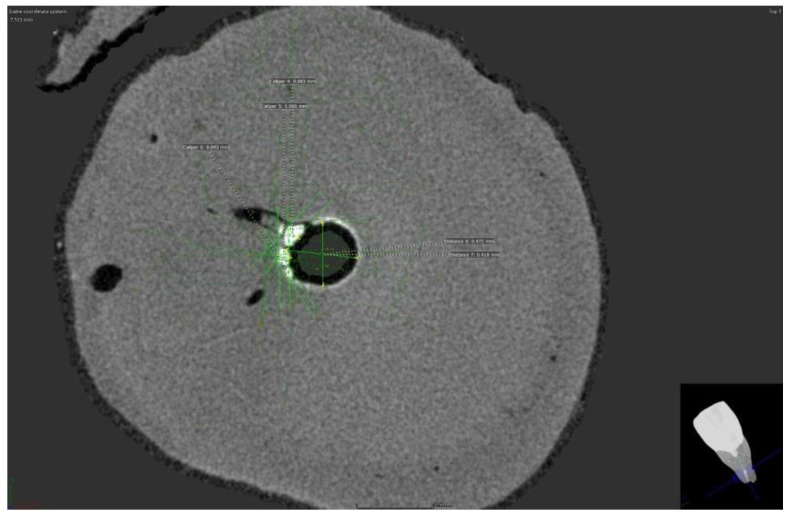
Micro CT scan of sample with preserved smear layer.
